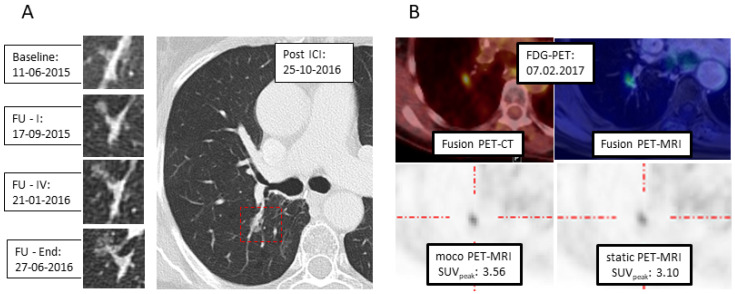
Example of respiratory motion correction in PET-MRI. (A) CT: Development of a semi-solid formation post-immunotherapy at the right hilum. (B) ^18^F-FDG-PET with PET/CT (upper left) und PET-MRI (upper right): With MRI-based motion correction (moco), a higher SUV_peak_ is calculated (lower left), compared to static reconstruction (lower right).
